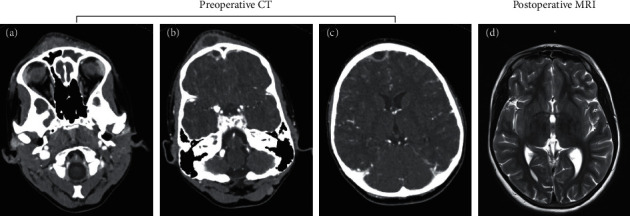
Imaging findings before and after balloon dilation. (a)–(c) Preoperative head CT slices with contrast of one case included in the study: (a) fluid-density opacification of the frontal sinus; (b) contrast-enhancing subperiosteal fluid collection overlying the frontal bone, consistent with Pott's puffy tumor; (b)-(c) contrast-enhancing intracranial fluid collection consistent with epidural abscess. (d) T2 MRI six weeks after surgery revealing resolution of abscesses and aeration of the frontal sinus.
